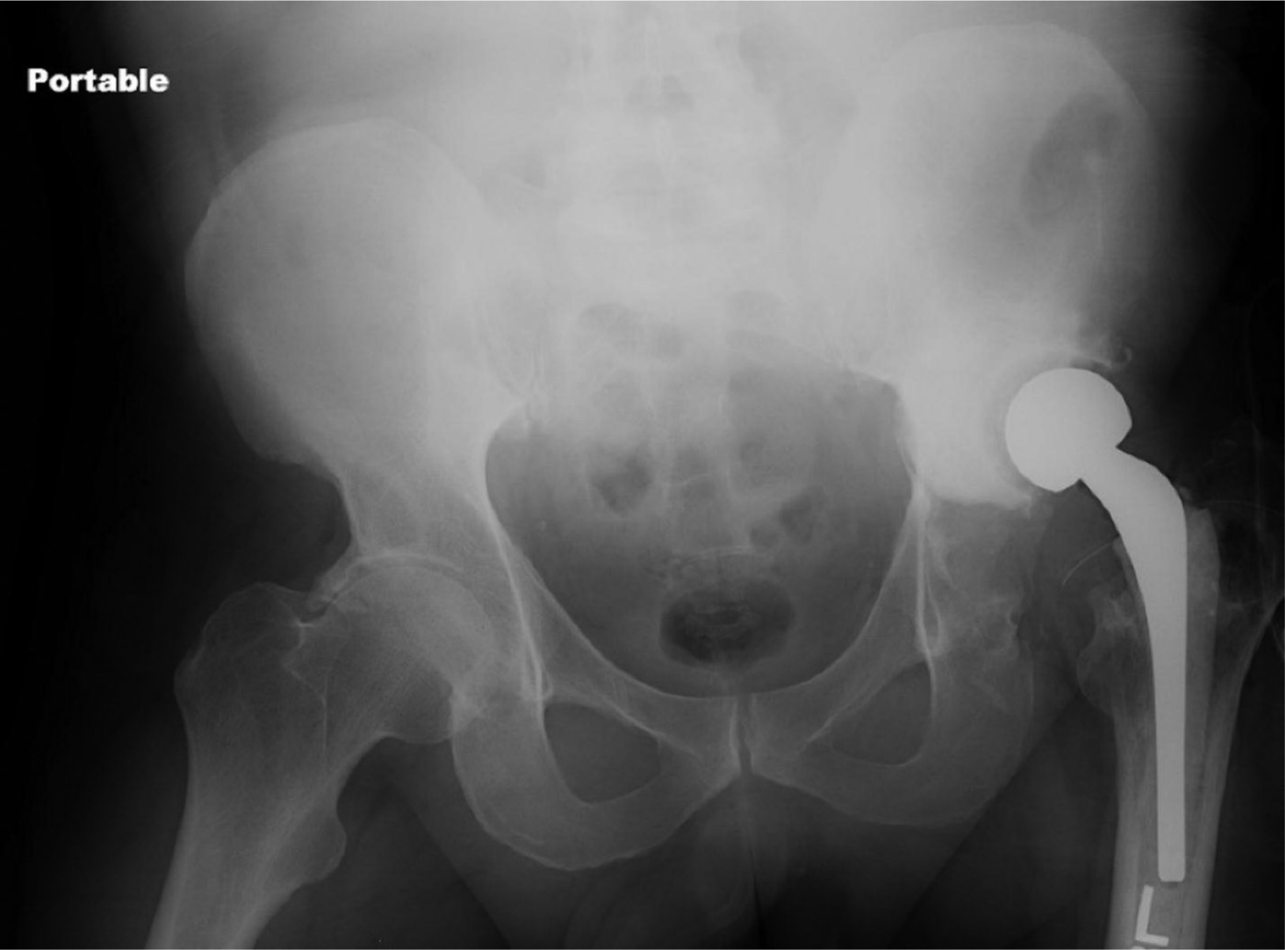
Anteroposterior radiograph of the pelvis showing a Prostalac spacer immediately following stage-1 revision.
